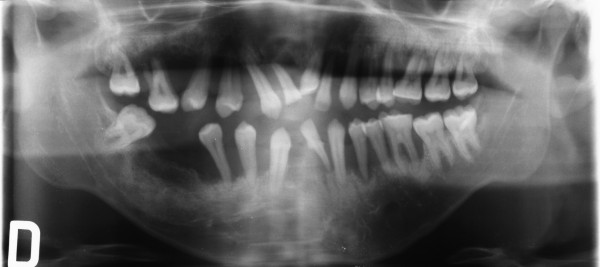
A panoramic X-ray of the same patient. An advanced generalised destruction of the supporting bone tissue is evident. One of the most important topics in periodontal diagnosis in the next future will be to create microfluidic chips allowing healthcare providers in poorly equipped hospitals and areas of the world.
